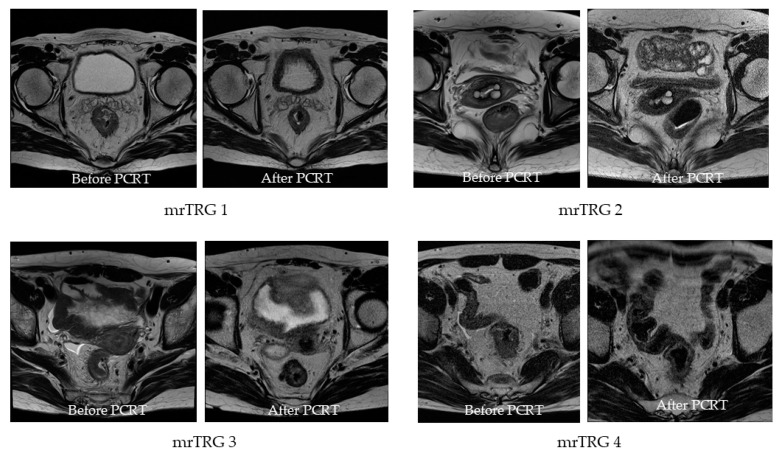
Tumor response after preoperative chemoradiotherapy (PCRT) with MRI as mrTRG. mrTRG, tumor regression grade evaluated with MRI; mrTRG1, complete regression; mrTRG2, near complete regression; mrTRG3, moderate regression; mrTRG4, minimal to no regression.
